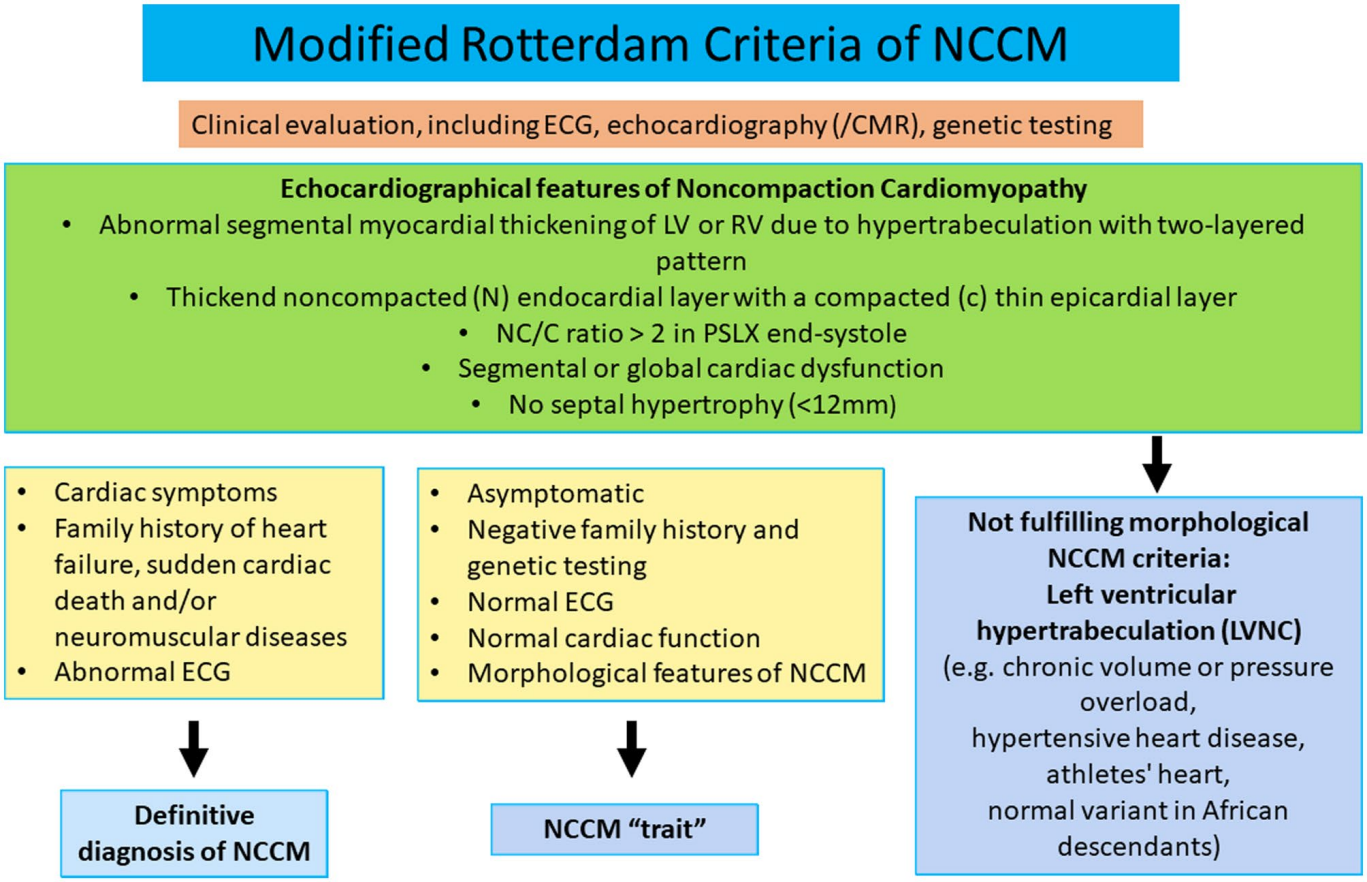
Diagnosing NCCM according to the Rotterdam criteria [18]. LVNC left ventricular noncompaction, NCCM noncompaction cardiomyopathy, NMD neuromuscular disease, PSLX parasternal long axis view
